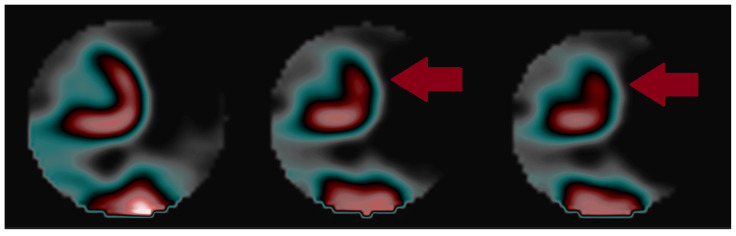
Performed SPECT showed slightly decreased flow at rest in the subapical segment (13), and at exercise after achieving 8.6 METs, a decrease in perfusion in a segment of the anterior wall (1 and 7) with a total flow decrease of 6% (mild reduction). The patient qualified for conservative treatment and observation.
